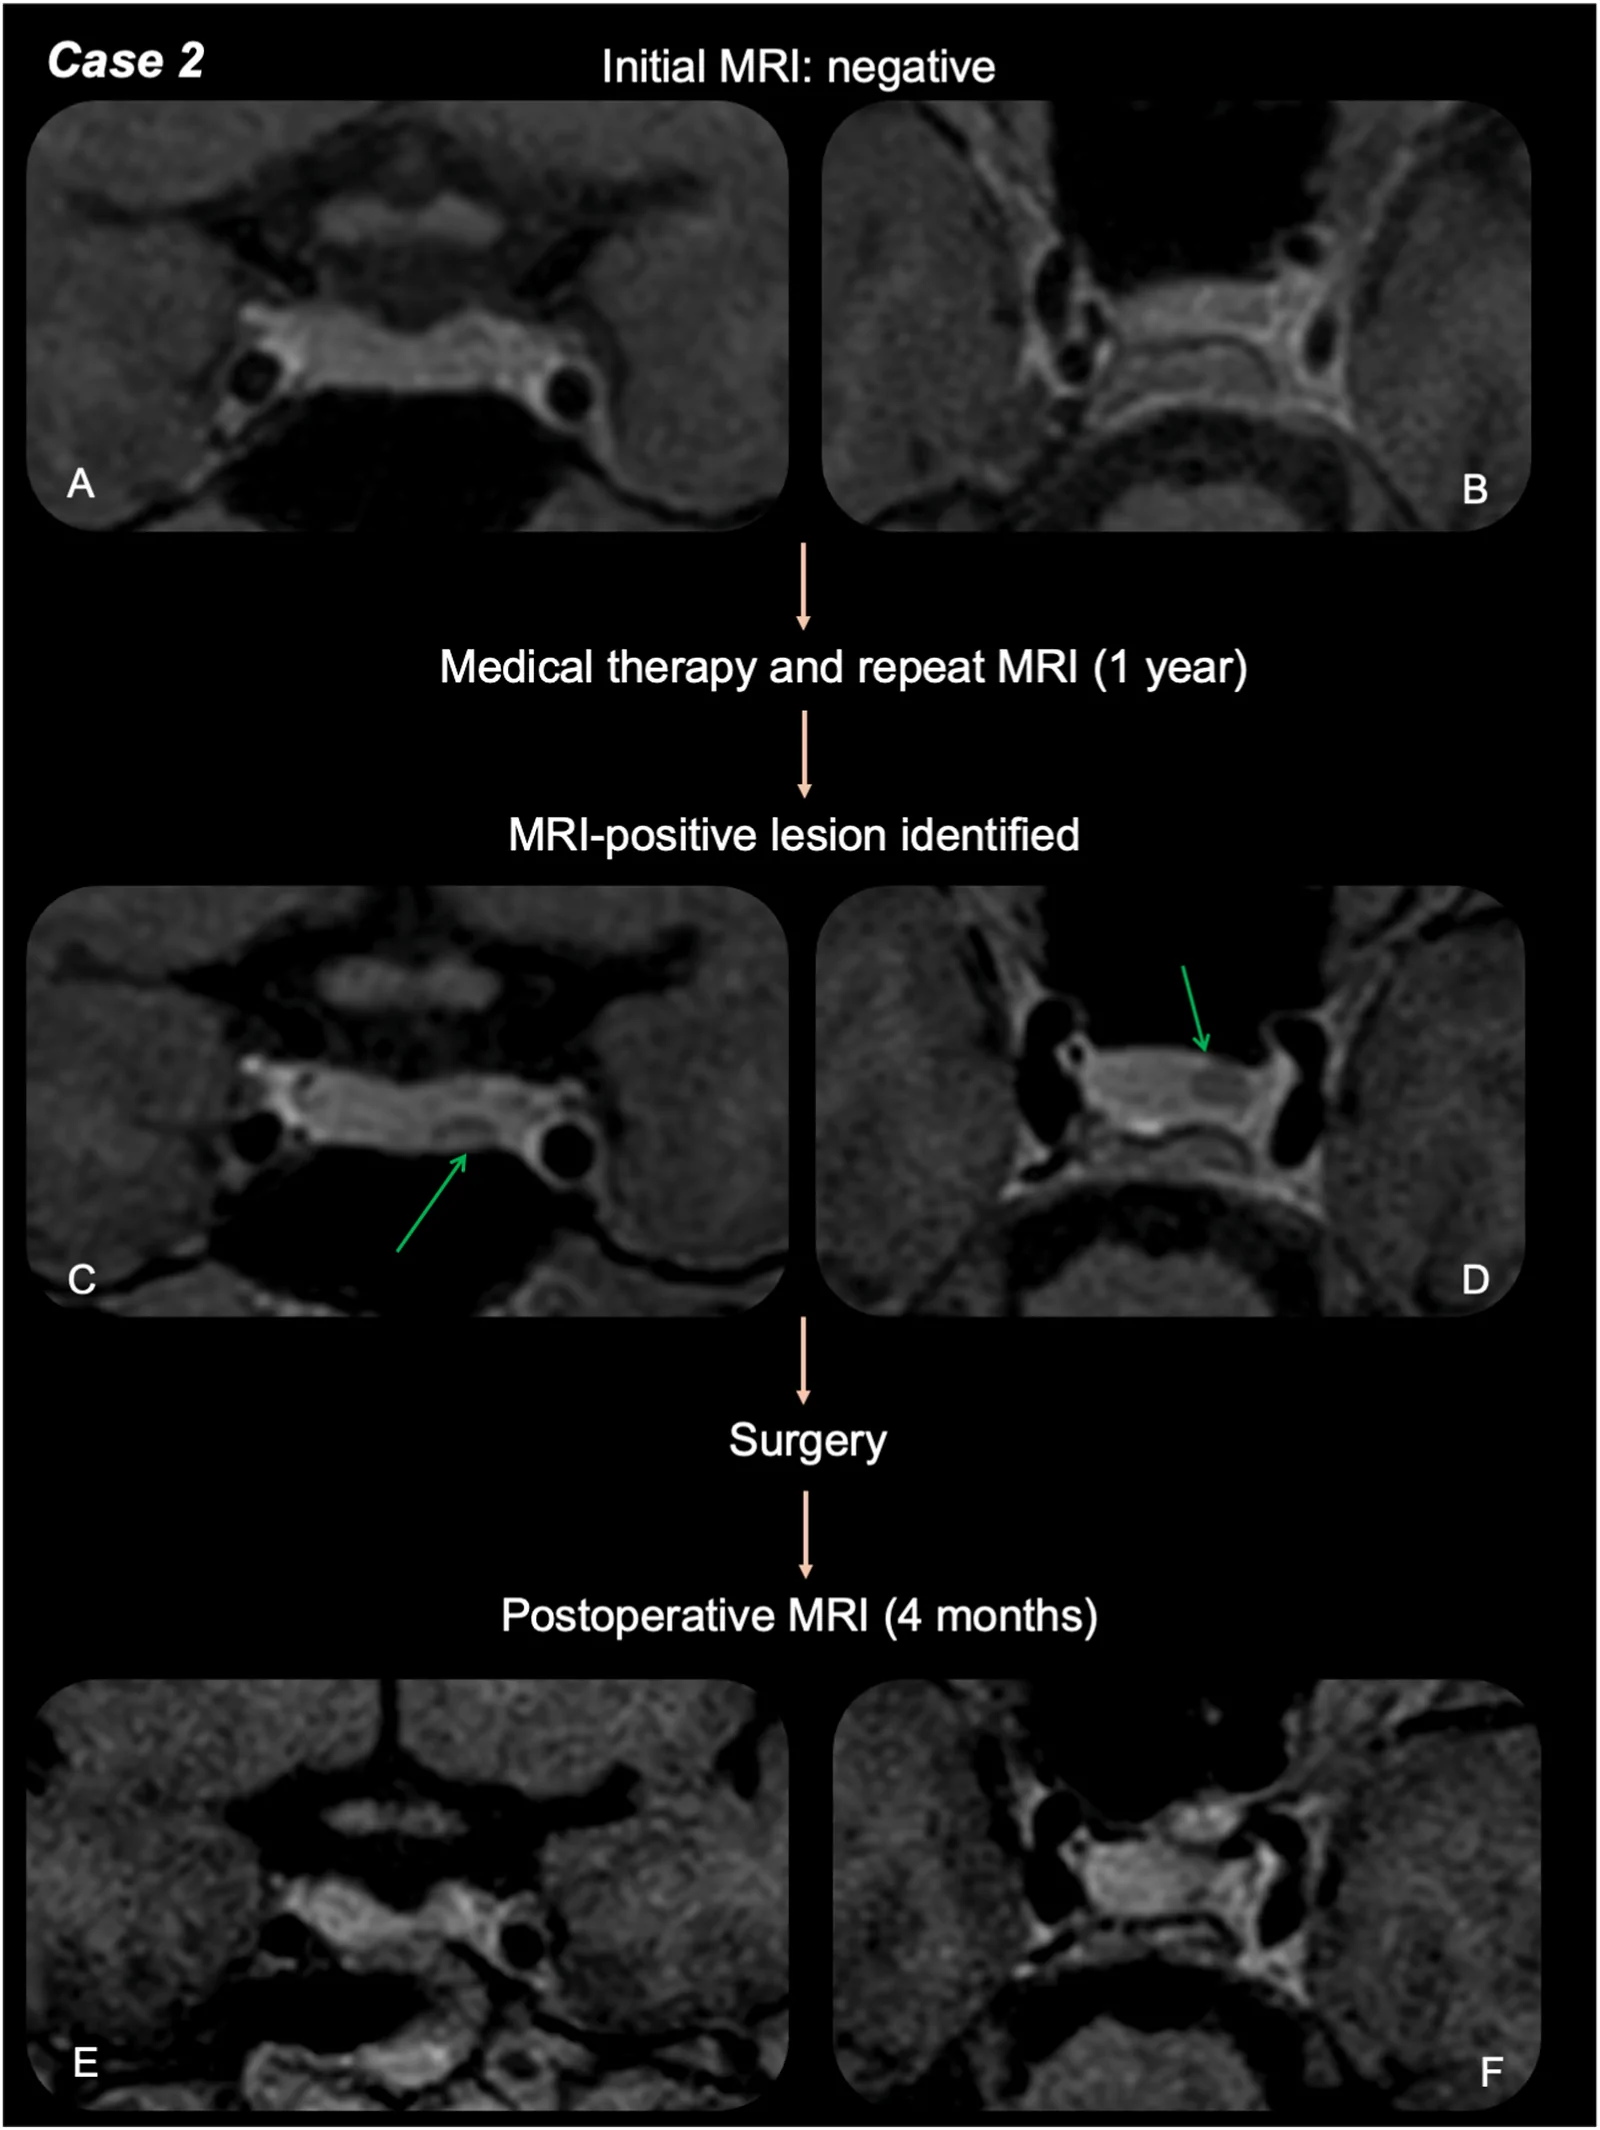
MRI-positive with a microadenoma and basal dura invasion (Case 2) (A–B) Initial coronal T1-weighted pituitary MRI showing no clear identifiable lesion. Following one year of medical therapy with osilodrostat and repeat imaging, a focal 6-mm left-sided lesion became visible. (C–D) Repeat MRI demonstrating a left-sided basal microadenoma (green arrows), consistent with an ACTH-secreting lesion. (E–F) Postoperative MRI at 4 months showing complete resection after endoscopic transsphenoidal adenomectomy with basal dura resection, with no residual tumor.
